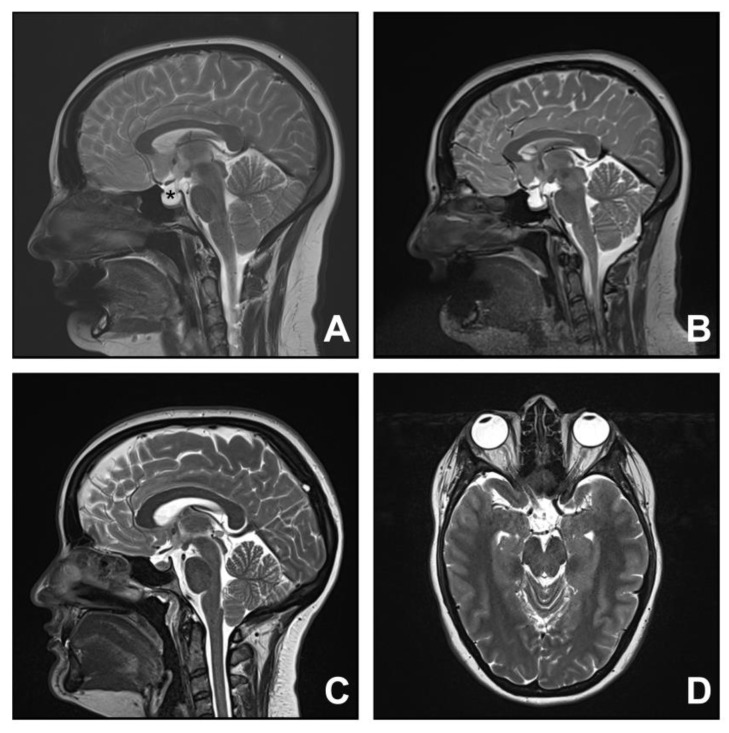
Chiari mimic: idiopathic intracranial hypertension. Two female patients with high body mass indexes (>30) (Case 11. (A,B); Case 12. (C,D)) presented with occipital headaches exacerbated by cough and MRI features suggestive of Chiari I malformation (A,C). In addition, there were concomitant imaging features of intracranial hypertension, such as empty sella ((A); * for empty sellar) and tortuosity and distension of the optic nerves (D). There was a persistent empty sella (B) post-posterior fossa decompression.
